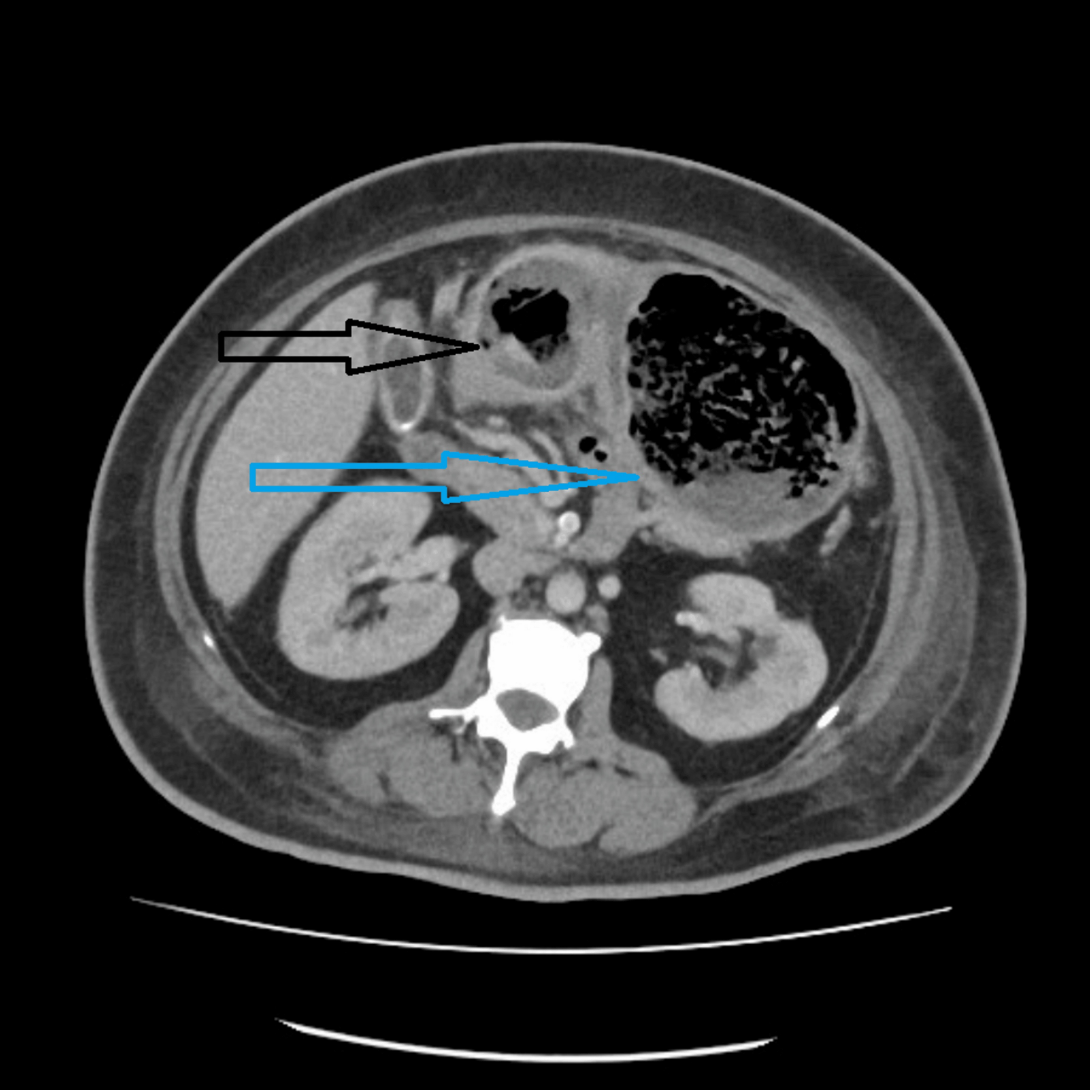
CT images detailing resolution of gastric body wall thickening (blue arrow) and pyloric intramural air hypodensities (black arrow).
